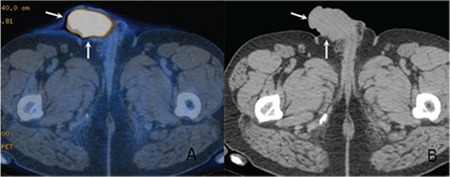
Transaxial fused positron emission tomography-computed tomography (A) and computed tomography (B) images showing the penile soft tissue mass with intense F-18 fluorodeoxyglucose uptake (arrows).
